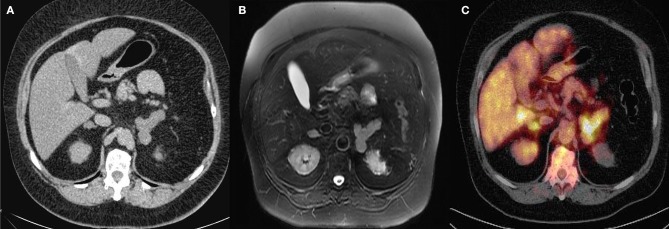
(A) Pre-contrast abdominal computed tomography (CT) scan (case # 3). (B) Post-contrast abdominal CT (C) Fluorine-18-fluorodeoxyglucose positron emission tomography 18F-FDG-PET/CT (case # 3) with Cushing's syndrome ACTH pituitary independent with bilateral adrenal nodules.
